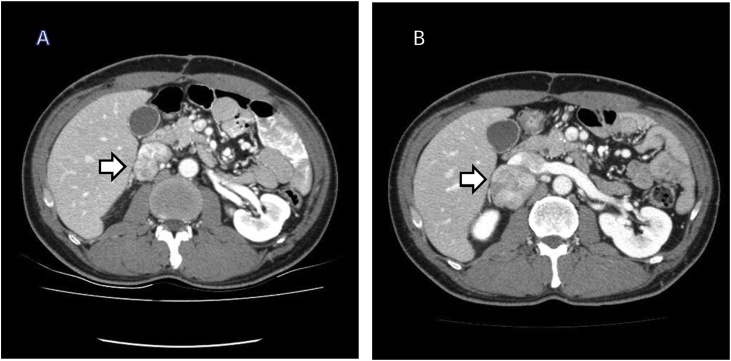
Computed tomography scan. Computed tomography scans showed an enlarged retroperitoneal lymph node of the posterior inferior vena cava (A) and rapidly enlarged lymph node after chemotherapy (B).
